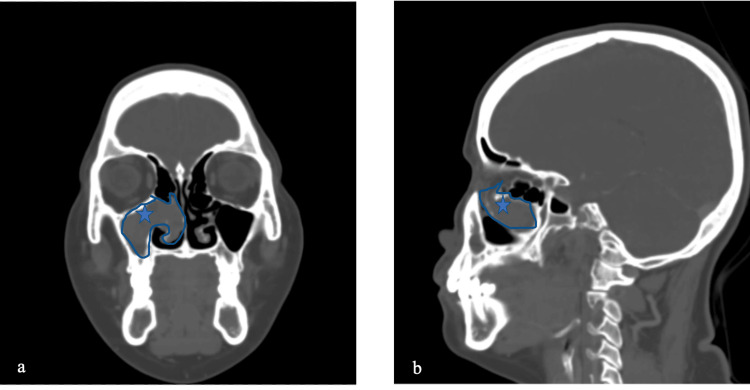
Preoperative contrast-enhanced computed tomography (CT) scan of the paranasal sinuses. (a) coronal view (bone window), and (b) sagittal view (bone window). The blue star indicates the tumor's pedicle of inverted papilloma.
